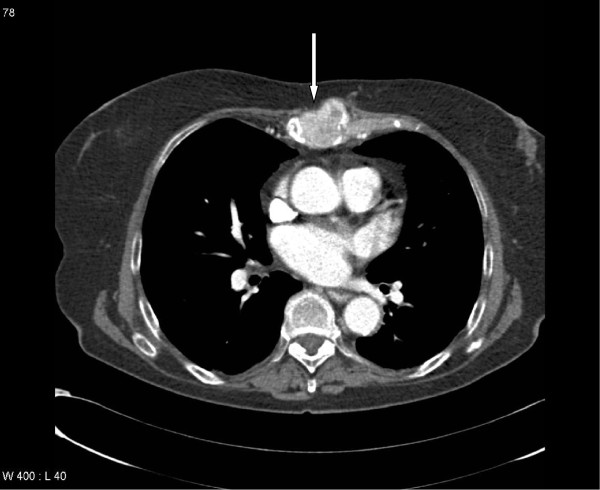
Computed tomography scan in a patient with clear cell kidney cancer and uncomplicated bone pain (bone lesions not causing neurological complaints and without a high risk of pathological fracture), extra-osseous extension present (white arrow), known lung and adrenal gland metastases: treated with 8 Gy x1.
